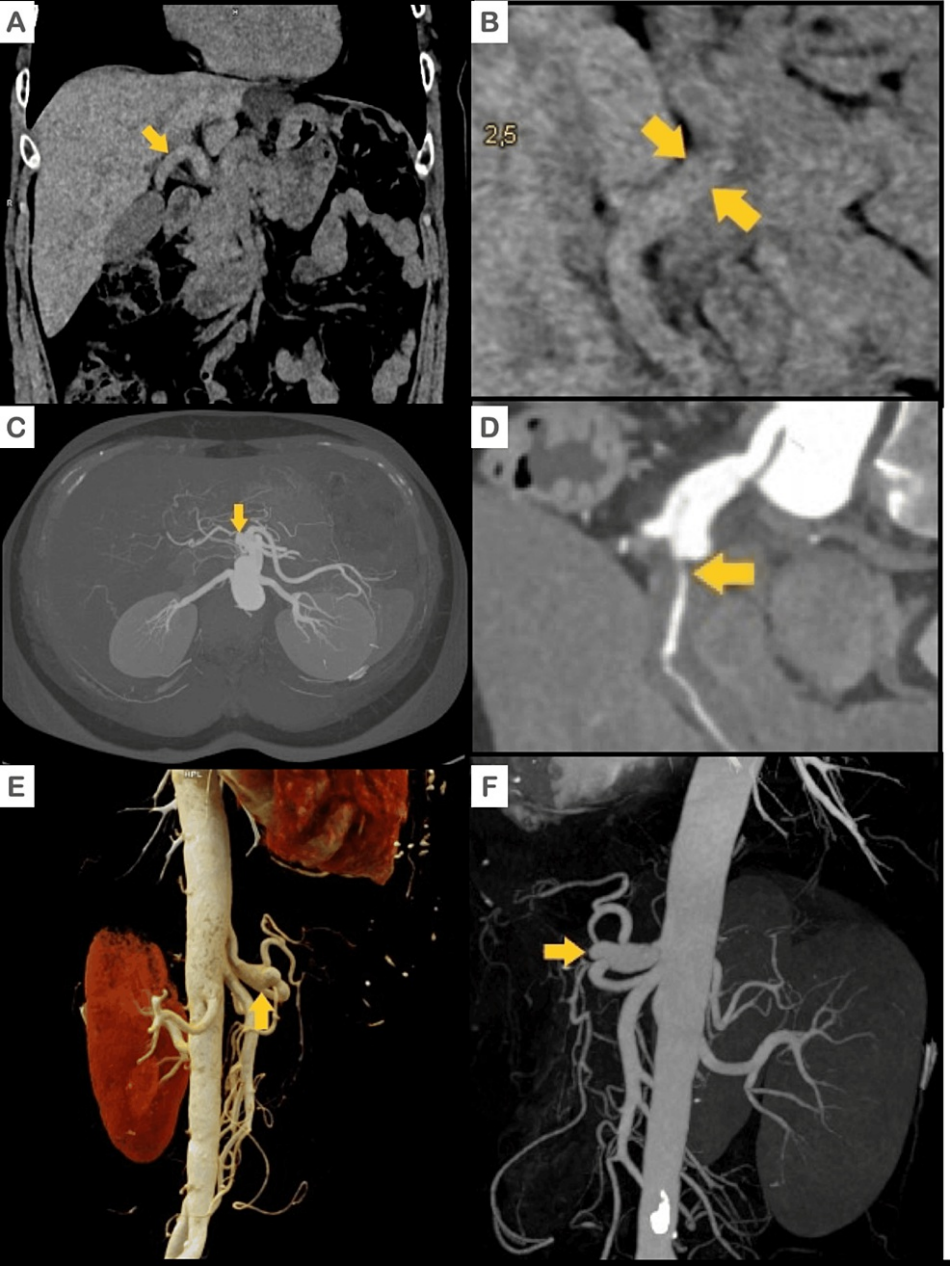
Abdominal CT: Simple, contrasted, and tridimensional reconstruction Simple CT scan of the abdomen. The common hepatic artery with a thickened soft tissue density wall (A: coronal; B: axial). C and D: Computed tomography (CT) of the abdomen revealing dilatation of the distal celiac trunk and minimal lumen of the common hepatic artery (yellow arrow). E and F: Angiotomography with three-dimensional reconstruction depicting a fusiform aneurysm of the celiac trunk and amputation of the common hepatic artery.
